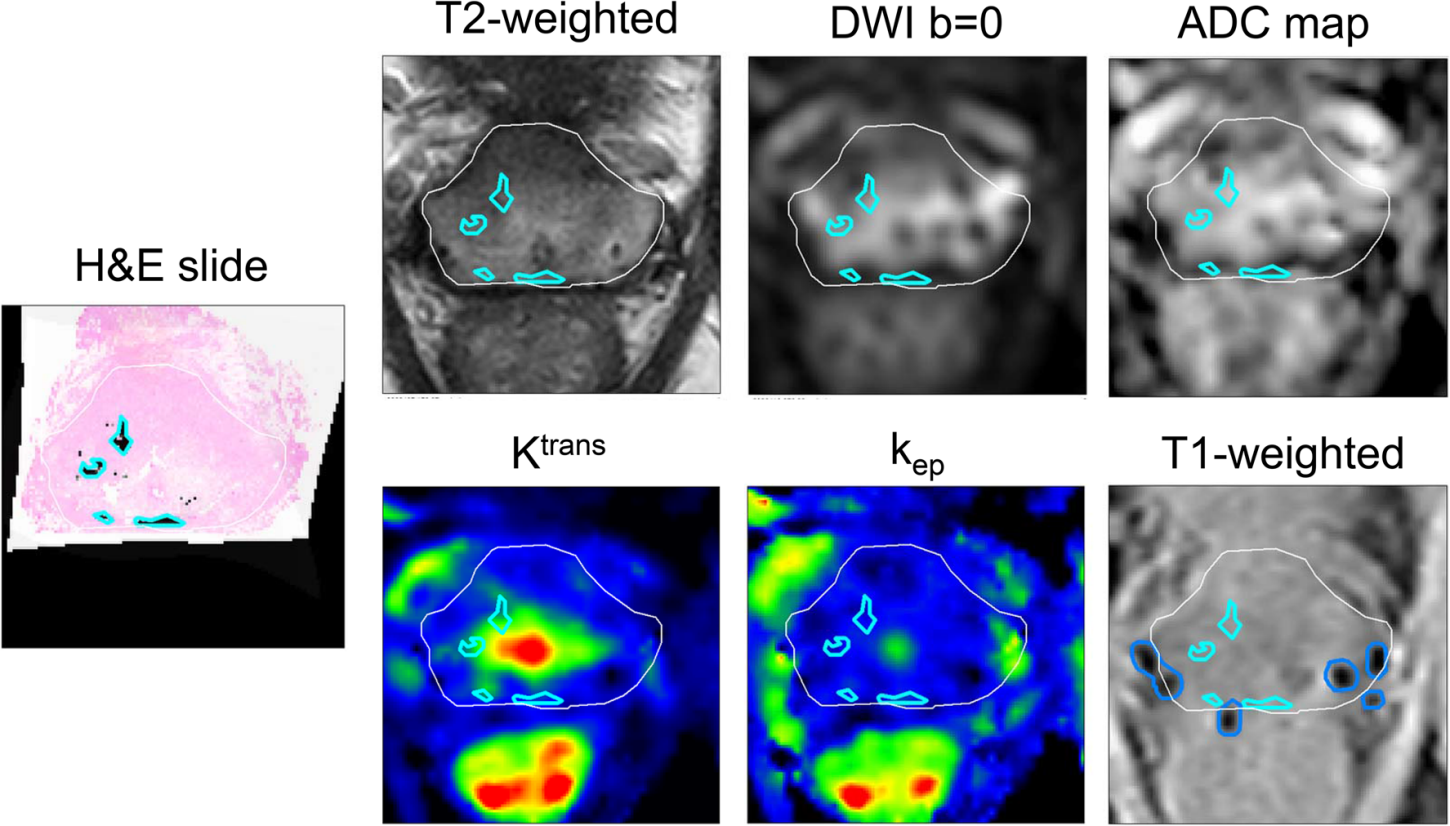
Example of a LDR brachytherapy patient in which the DWI sequence is distorted by the presence of the seeds. The region masked out of the analysis due to the presence of the seeds (in dark blue) was delineated using the T1w gradient echo sequence
